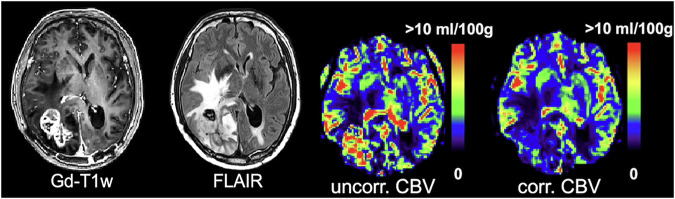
Patient with brain metastasis from anaplastic lymphoma kinase-mutated non–small-cell lung cancer. Anatomical images (Gd-T1w, FLAIR) together with uncorrected and corrected CBV. Note that leakage correction strongly reduced the elevated uncorrected CBV values in the lesion area. CBV values were normalized by setting white matter to 2 mL/100 g. Figure parts reproduced from Knutsson et al [48] under the terms of the Creative Commons Attribution License
